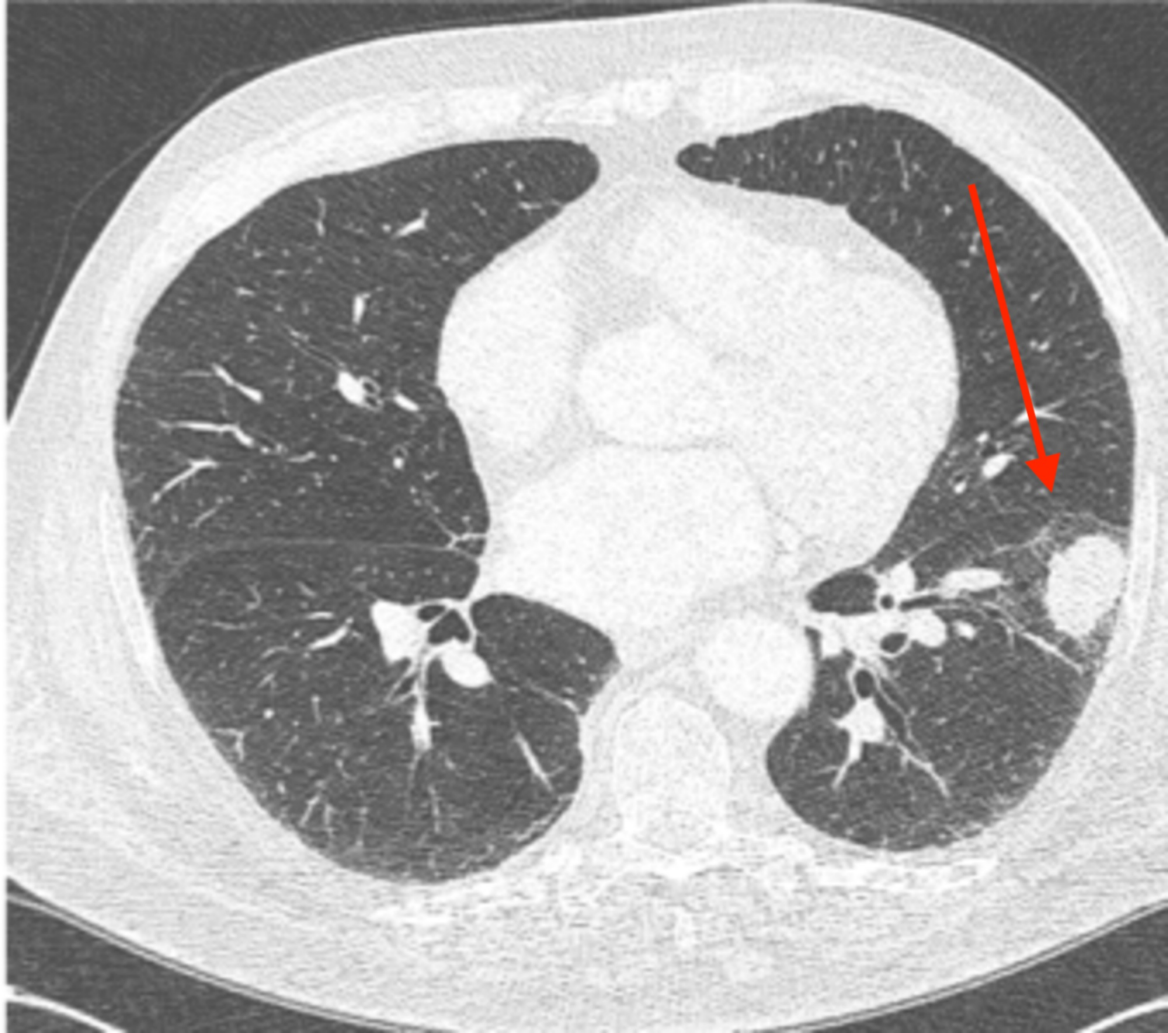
The axial slice from the baseline computed tomography (CT) chest is shown measuring 3.2 x 2.2 cm
